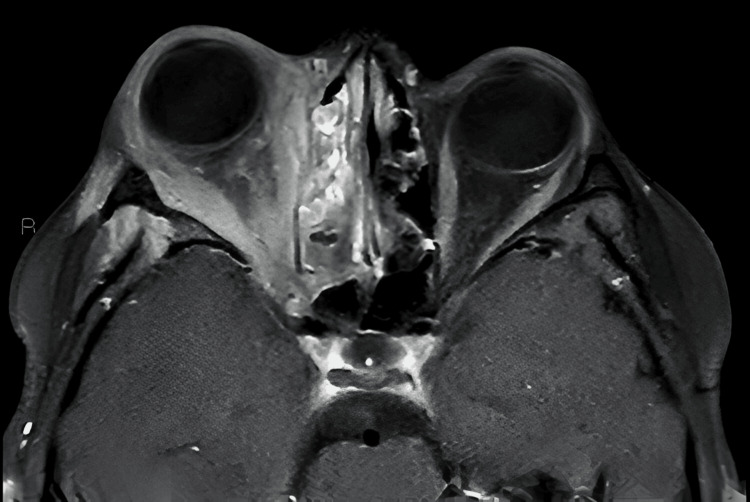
Post-contrast T1-weighted MRI of the Brain demonstrating right-sided proptosis with extensive periorbital and orbital inflammation
